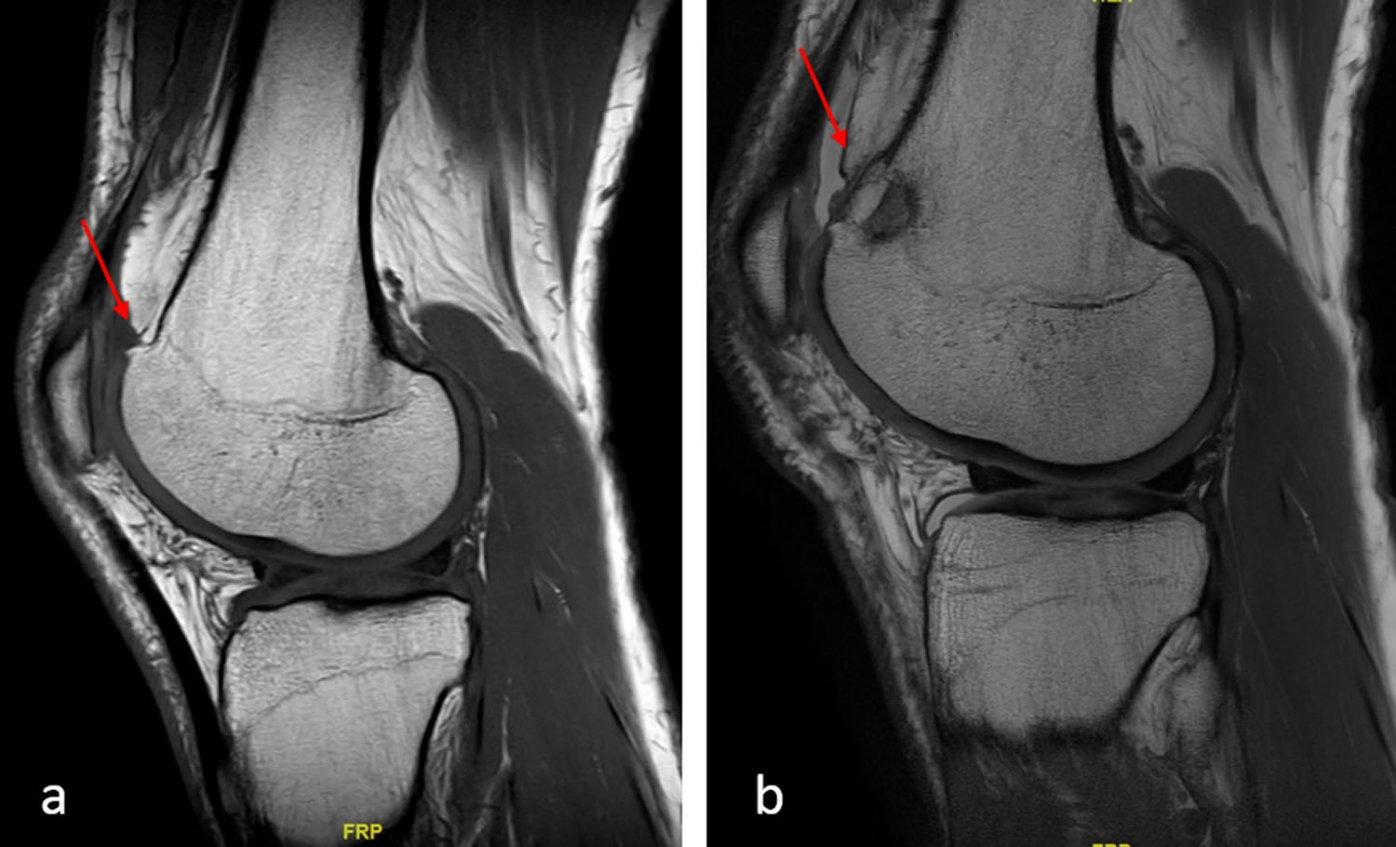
Preoperative (a) and postoperative (b) MRI images of a lateral trochlear lengthening osteotomy in a patient with short lateral trochlea and presence of a lateral bump (red arrow, a). Note the lengthening of the trochlea and the leveling of the bump postoperatively (red arrow, b)
